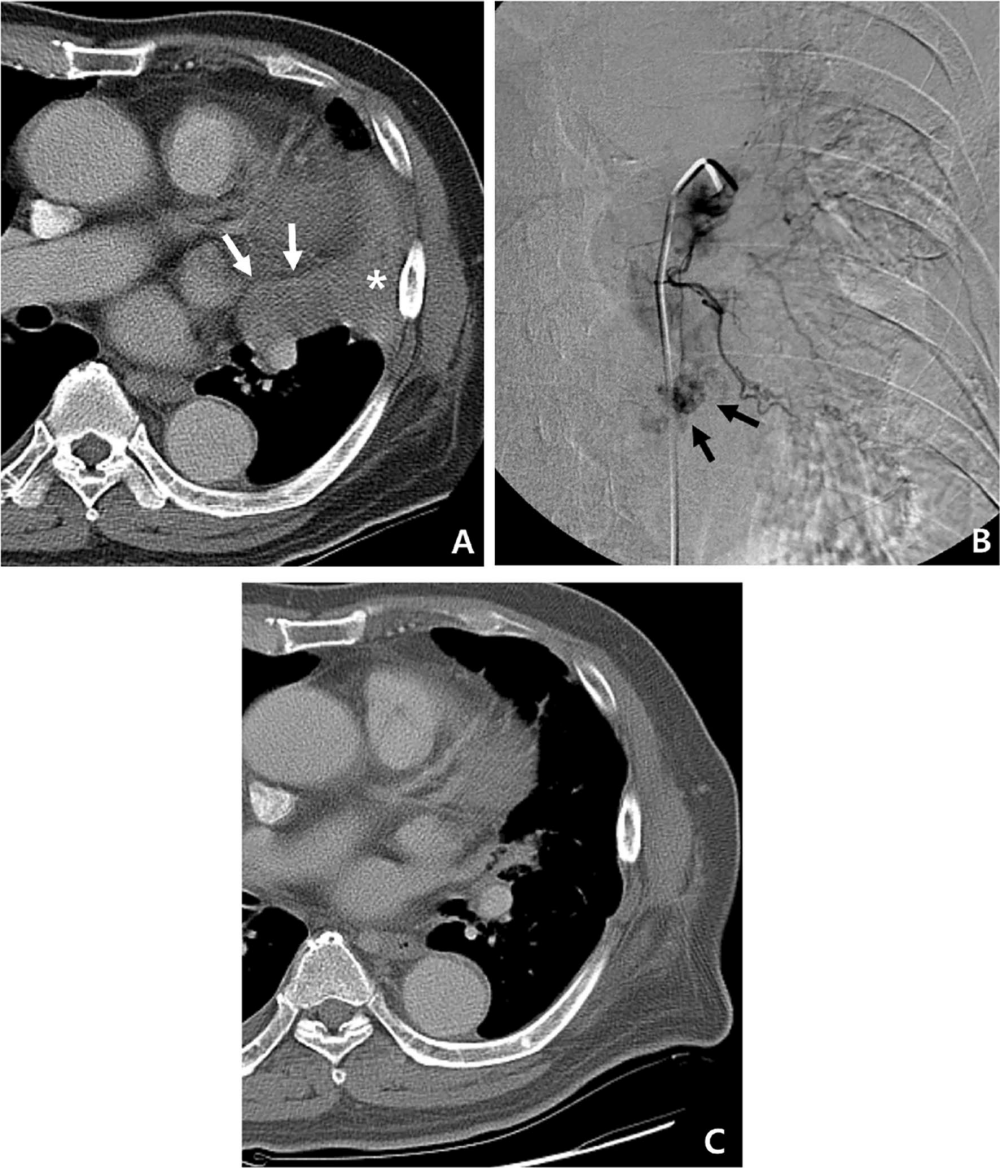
An 81-year-old man with pulmonary metastasis from HCC who presented with a moderate amount of hemoptysis. (A) Axial CT image before embolization shows obstructive pneumonitis (asterisk) in the left upper lobe lingular division caused by a metastatic mass (white arrows). (B) Selective left bronchial arteriography shows a tortuous hypertrophied left bronchial artery with a hypervascular tumor blush (black arrows) in the proximal portion of the lingular division. Embolization of the left bronchial artery was successfully performed using gelfoam, and this patient showed complete resolution of hemoptysis following bronchial artery embolization. (C) Axial CT image 4 months after embolization of the bronchial artery shows the metastatic mass in the lingular division to be markedly decreased in size, as well as an improvement in obstructive pneumonitis.
